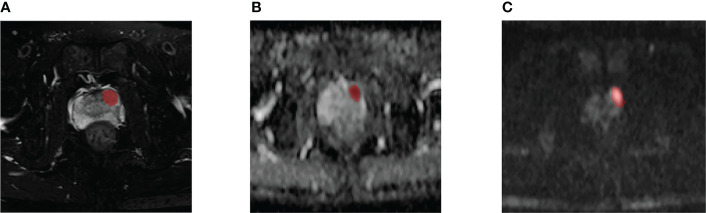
A 59-year-old man diagnosed with csPCa in PZ (FPSA, 0.04 ng/mL; TPSA, 4.27 ng/mL; biopsy GS, 4 + 4 = 8). Example segmentations (red masks) of the tumor overlaid on axial T2-weighted fat-sat imaging (T2WI-fs) (A), apparent diffusion coefficient (ADC) map (B), and diffusion-weighted imaging (DWI) (C).
